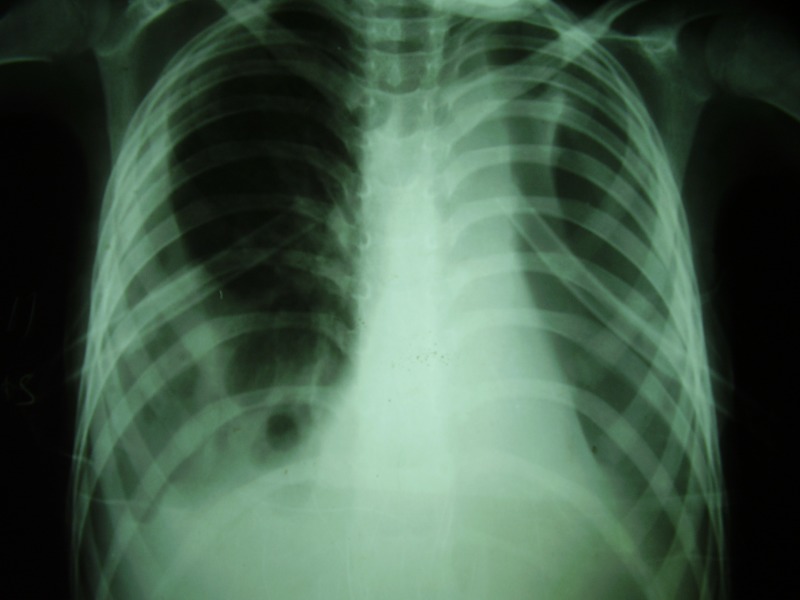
Figure 3: Post intubation chest X-ray.
